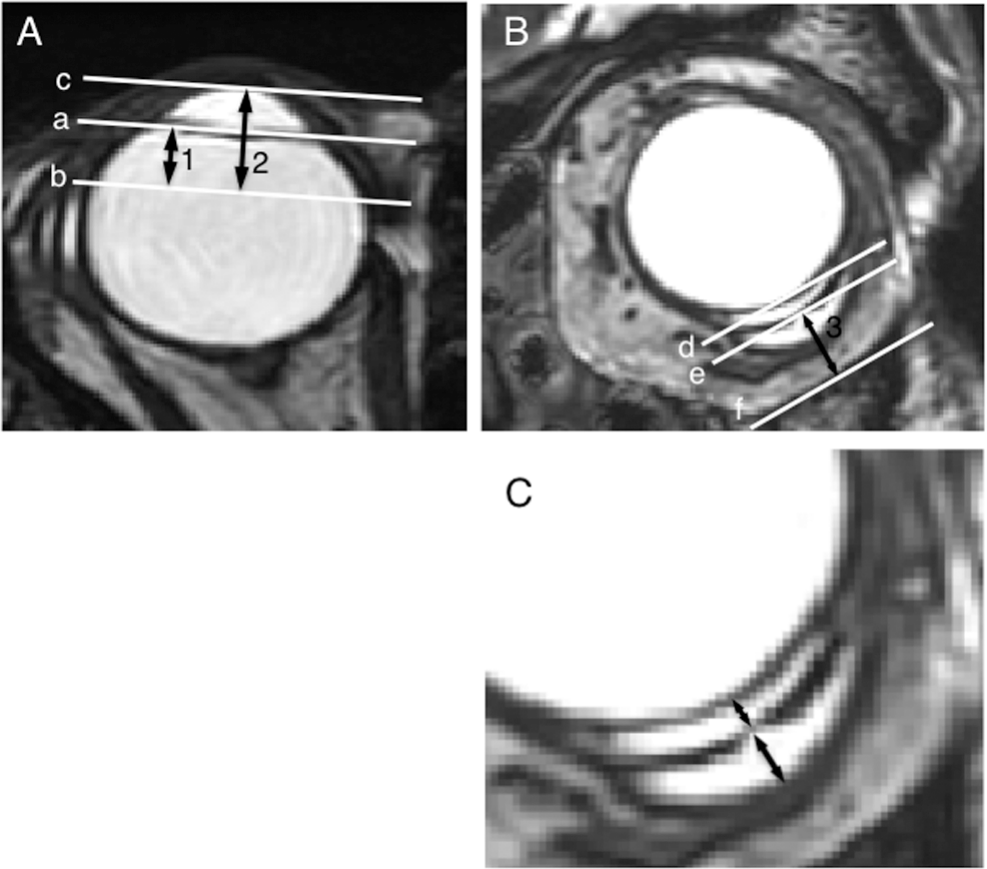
Definitions of magnetic resonance imaging-measured parameters. (A) Line a connects both edges of the corneal limbus on the axial scan image at the level of the anterior edge of the shunt is seen most anteriorly. Line b passes the anterior edge of the endplate and is parallel to line a. Line c passes the inner surface of the central cornea and is parallel to line a. The number 1 indicates the distance between lines a and b, and 2 indicates the distance between lines b and c. (B) Line d connects both edges of the endplate on the coronal scan image at the level of the width of the endplate seen is the longest. Line e passes the center of the endplate and is parallel to line d. Line f is parallel to line d and passes the nearest point from line d to the orbital bony wall. The number 3 indicates the distance between lines e and f. (C) The bleb thickness is the sum of the inner and outer bleb heights measured at the midline of the endplate on the same image used for the distance 3 measurements.
